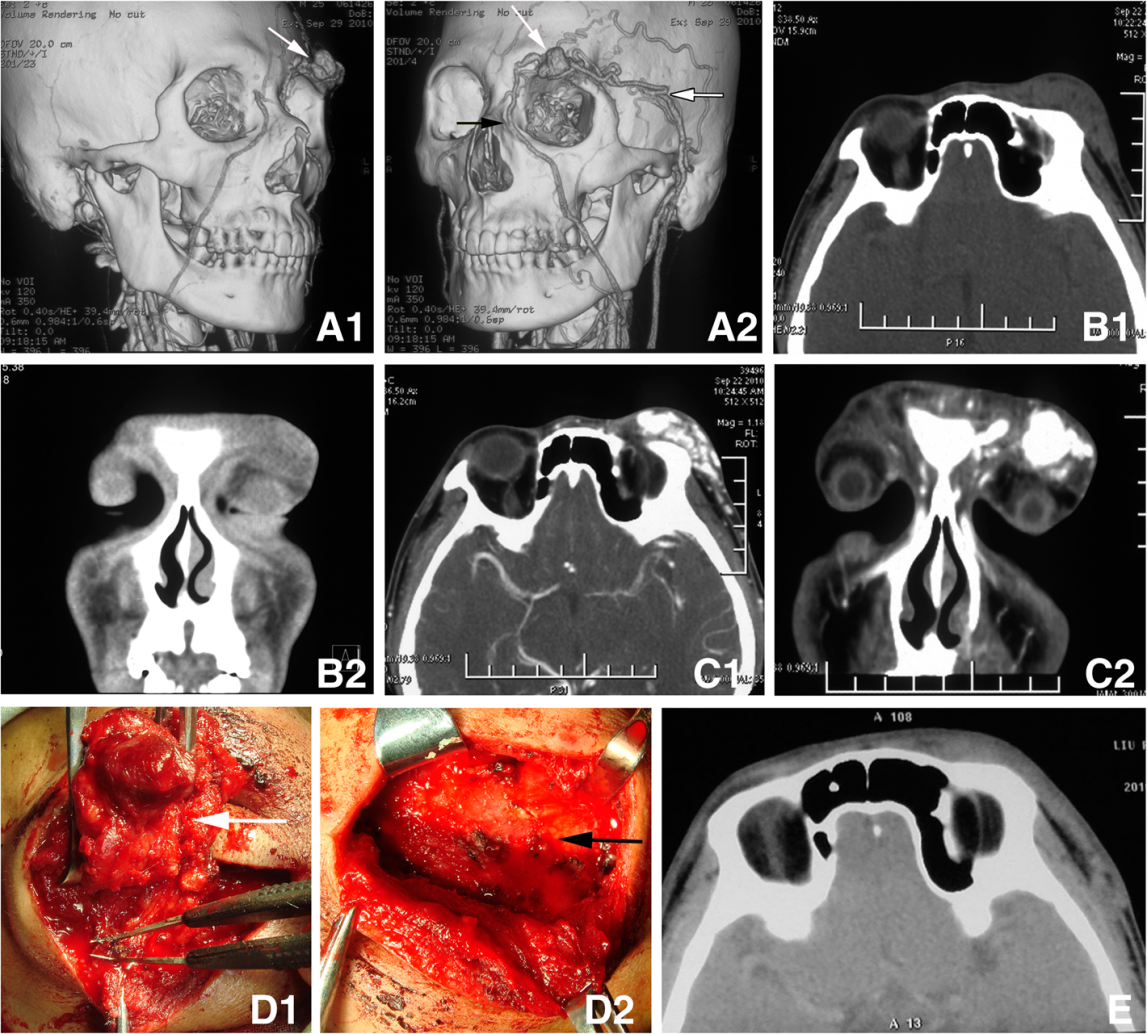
The 2nd case of local skin adverse effect *A* Three-dimensional computed tomographic(CT) angiography showed an abnormally expanded blood vessel mass (white arrow) in the soft tissue above the left orbit, supplied by the left superficial temporal artery, temporal artery (white arrow with dark outline) and arteria angularis (black arrow). The malformed blood vessel was not adjacent to the eyeball. *B*. CT imaging showed subcutaneous soft tissue mass in the upper left eyelid and forehead. *C*. Enhanced CT scan showed the vessel mass significantly heterogenous contrast enhancement. *D*. Surgical debulking was performed via a skin incision below the supraorbital ridge. White arrow indicates the solidified vascular malformation after medical glue injection. Black arrow indicates the exposed frontal bone after complete resection of the malformed blood vessel. *E*. CT scan showed the skin in the lateral-superior region of left orbit was slightly thickened at three months after surgery. Comparison of the pre-operation imaging the malformed blood vessel lesion was absent
